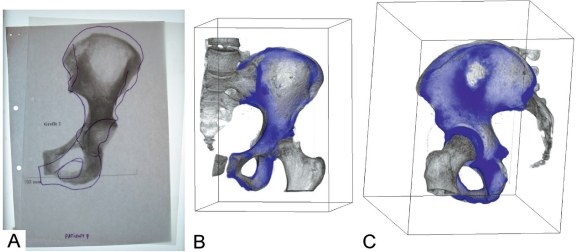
A. An example of the template method. It consists of a comparison between a fluoroscopy and a manually drawn template from an anteroposterior radiography of the recipient. B and C. Example of registration method (3-D views). The user can rotate the 3-D view to examine bone congruency. 2-D slices can also be inspected.
